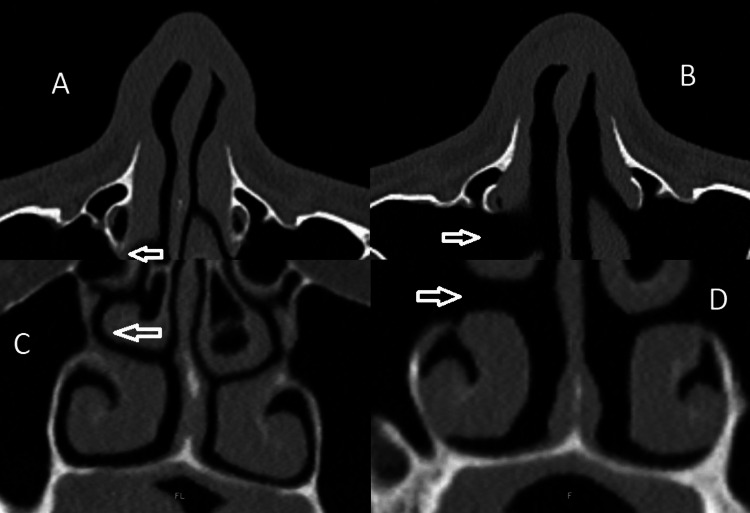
CT scan comparative status before and after PT with LNWL. A: Preoperative view in the axial cut of paranasal sinus CT scan. B: Status after PT and LNWL in the axial cut of paranasal sinus CT scan. C: Preoperative view in the coronal cut of paranasal sinus CT scan. D: Status after PT and LNWL in the coronal cut of paranasal sinus CT scan. CT = computed tomography; PT = pyriform turbinoplasty; LNWL = lateral nasal wall lateralization
